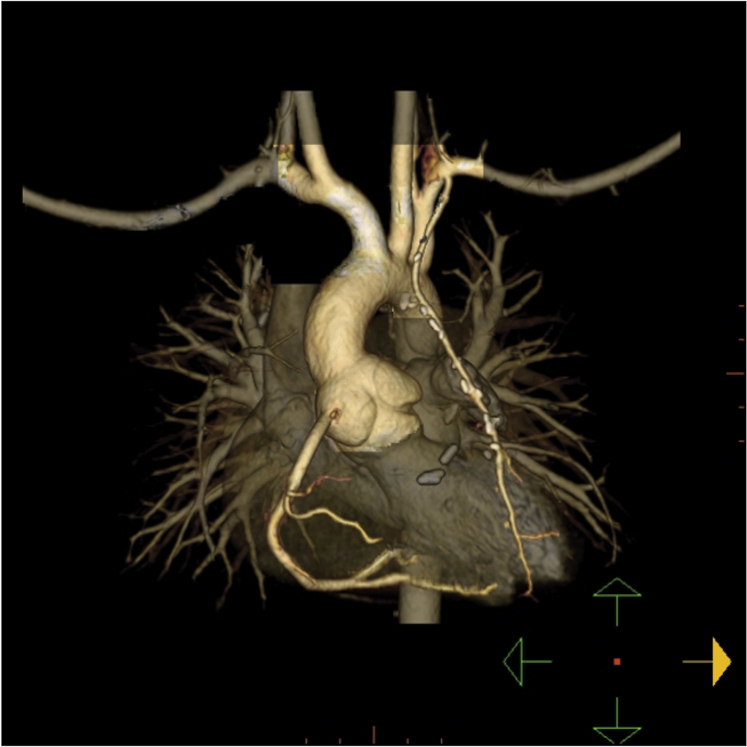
Postoperative cardiac computed tomography angiography with three-dimensional volume-rendered display performed 6 months postoperatively demonstrates a patent LIMA graft filling the LAD artery.
